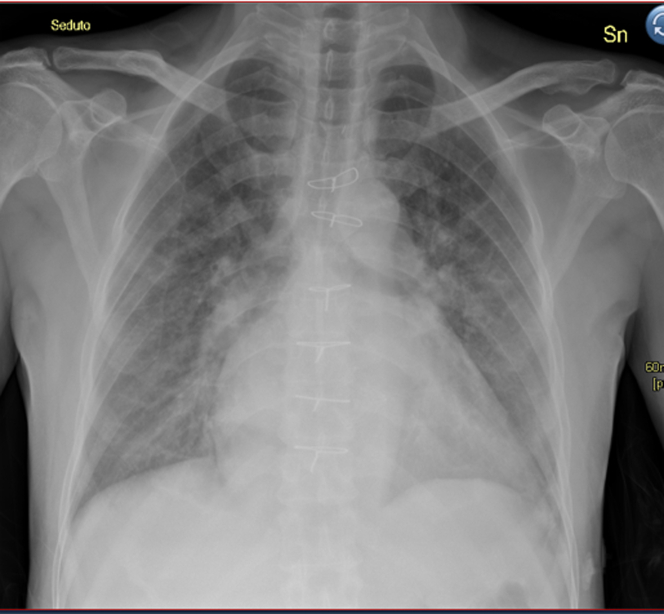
Chest X-Ray at Arrival in Emergency Department At the admission, the chest x-ray showed mild pulmonary congestion.
